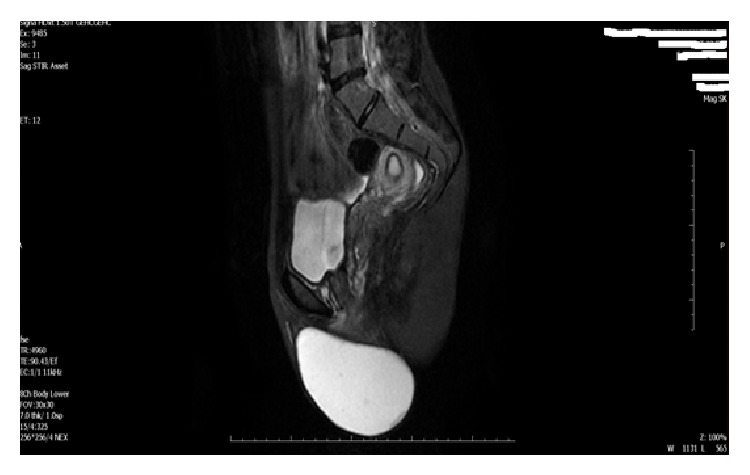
MRI image of 11 × 8 × 10 cm cystic mass with well-defined margins and without contrast enhancement.
